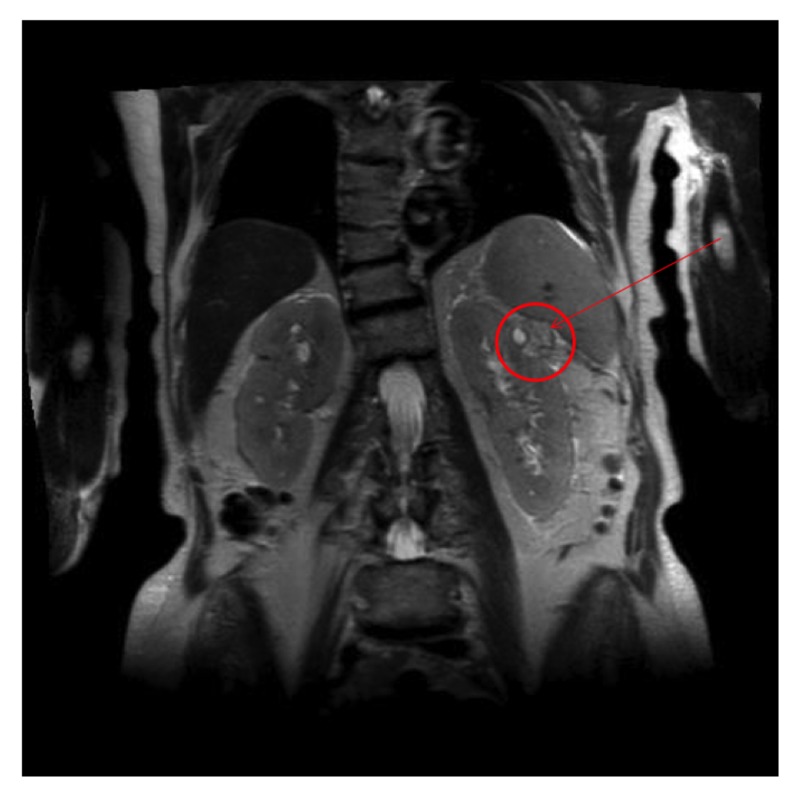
MRI of the kidneys The highlighted renal mass was biopsied and proven to be renal cell carcinoma. MRI: magnetic resonance imaging
